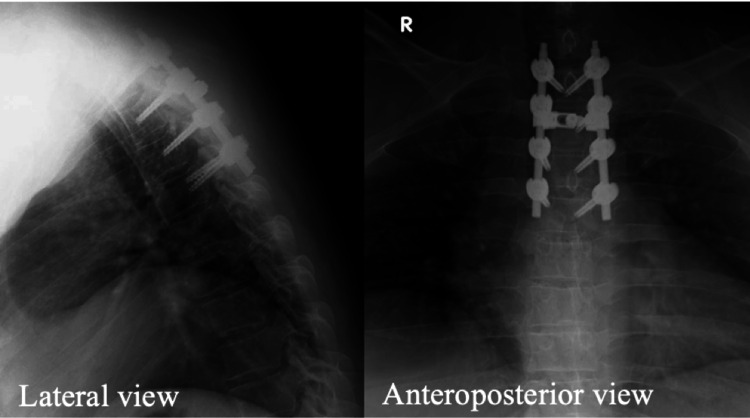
Anteroposterior and lateral plain radiography one week after surgery
